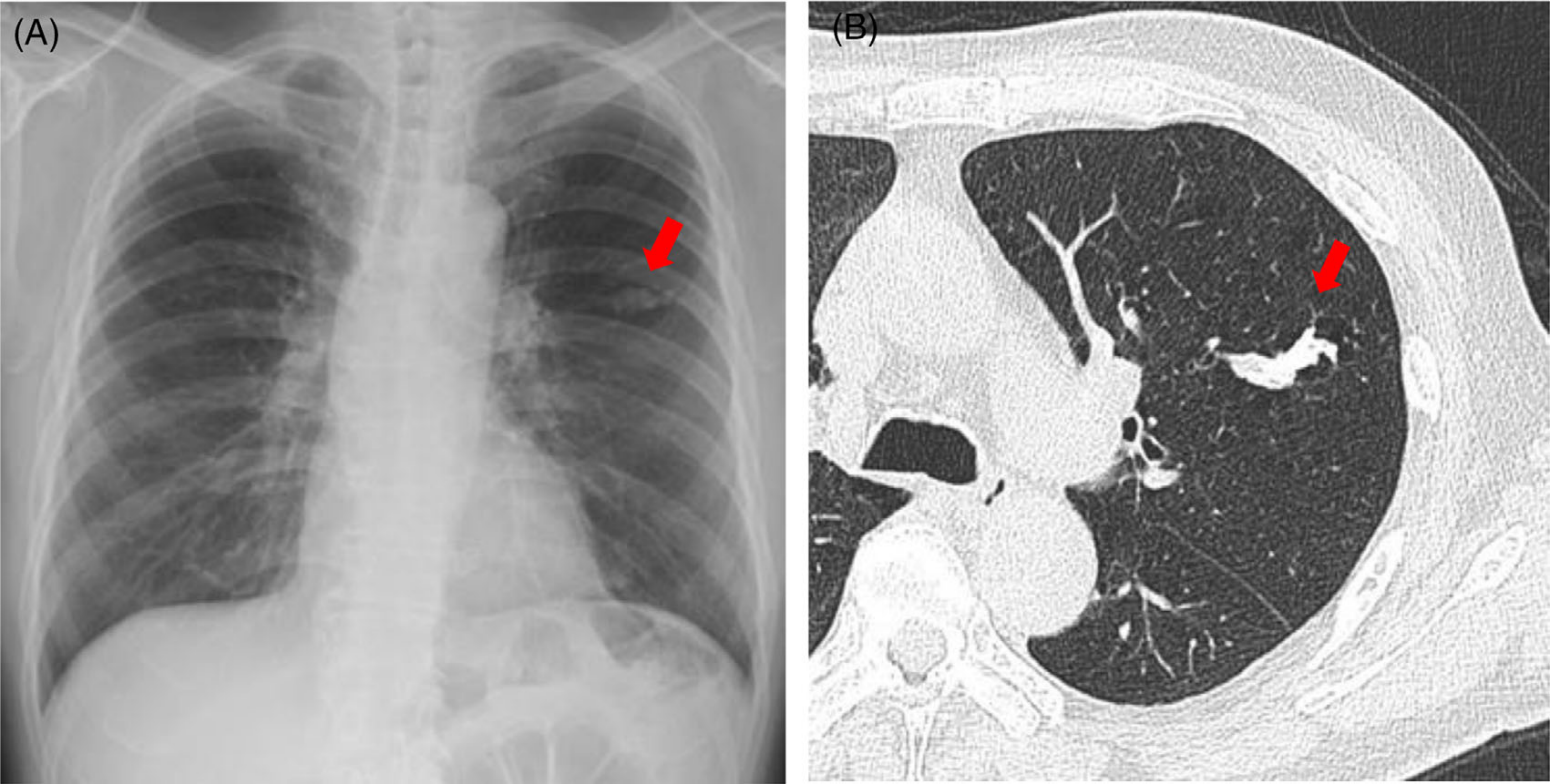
(A) Chest X‐ray showing a spindle‐shaped calcification in the left middle lung field; (B) chest computed tomography (CT) scan showing calcification along the left B3a
